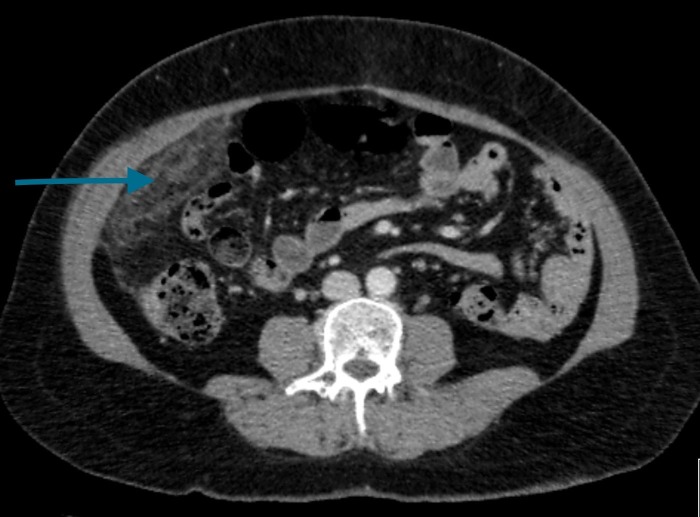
Computed tomography scan - axial view demonstrating fat stranding around the greater omentum.
